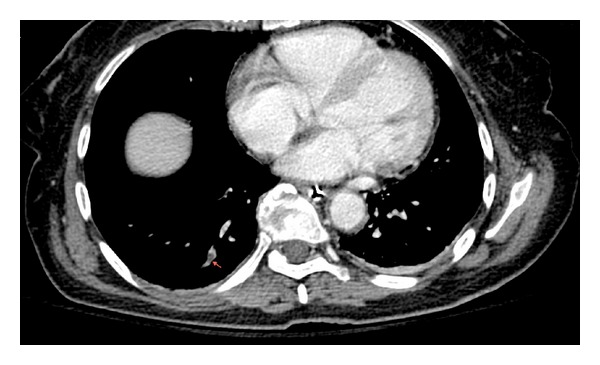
Computed tomography showing right subsegmentary pulmonary embolism (arrows).
